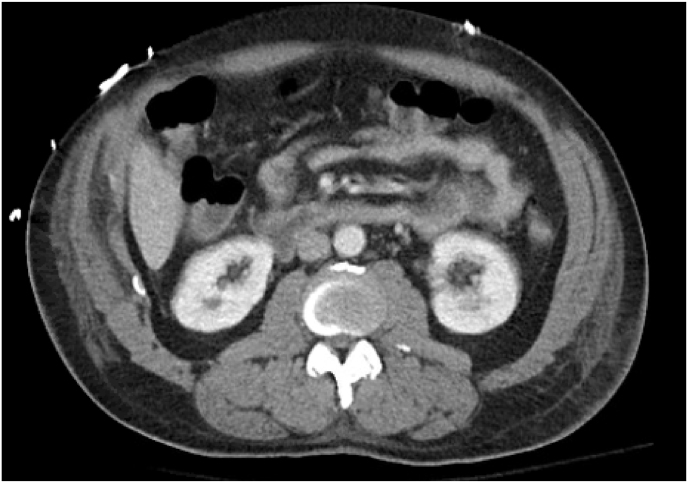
CT image of the abdomen with bilateral wedge-shaped renal infarcts.
